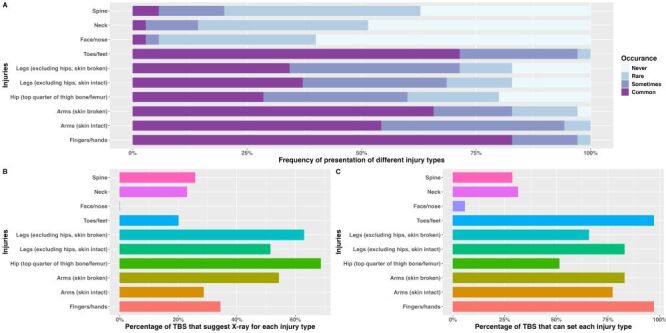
Traditional bone-setter care by type of injury. Panel A. Frequency of presentation of different injury types. Panel B. Percentage of TBS that suggested X-ray for each injury type. Panel C. Percentage of TBS that can set each injury type. TBS – traditional bone-setter.
